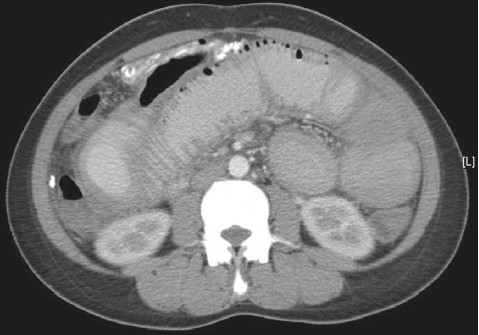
CT scan shows multiple dilated small bowel loops with multiple calcific peritoneal deposits.
